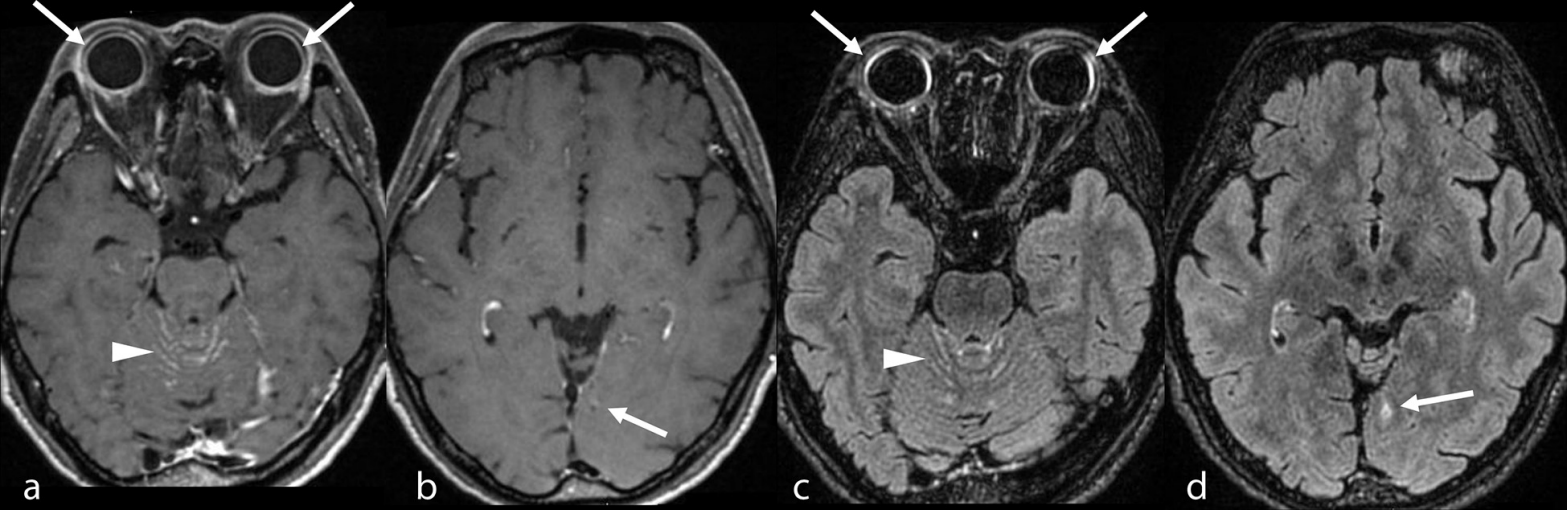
Central nervous system findings on MRI. Gd-enhanced 3D *T*_1_ weighted image shows abnormal enhancement in the bilateral choroid (arrows in a), bilateral cerebellar fissures (arrowhead in a) and leptomeninges of the left occipital lobe (arrow in b). Gd-enhanced 3D fluid attenuation inversion recovery images reveal a more conspicuous enhancement in the bilateral choroid (arrows in c) and leptomeninges of the left occipital lobes (arrow in d) compared with Gd-enhanced 3D *T*_1_ weighted image, although cerebellar fissure enhancement is similar to Gd-enhanced 3D *T*_1_ weighted image (arrowhead in c). Gd, gadolinium; 3D, three-dimensional.
